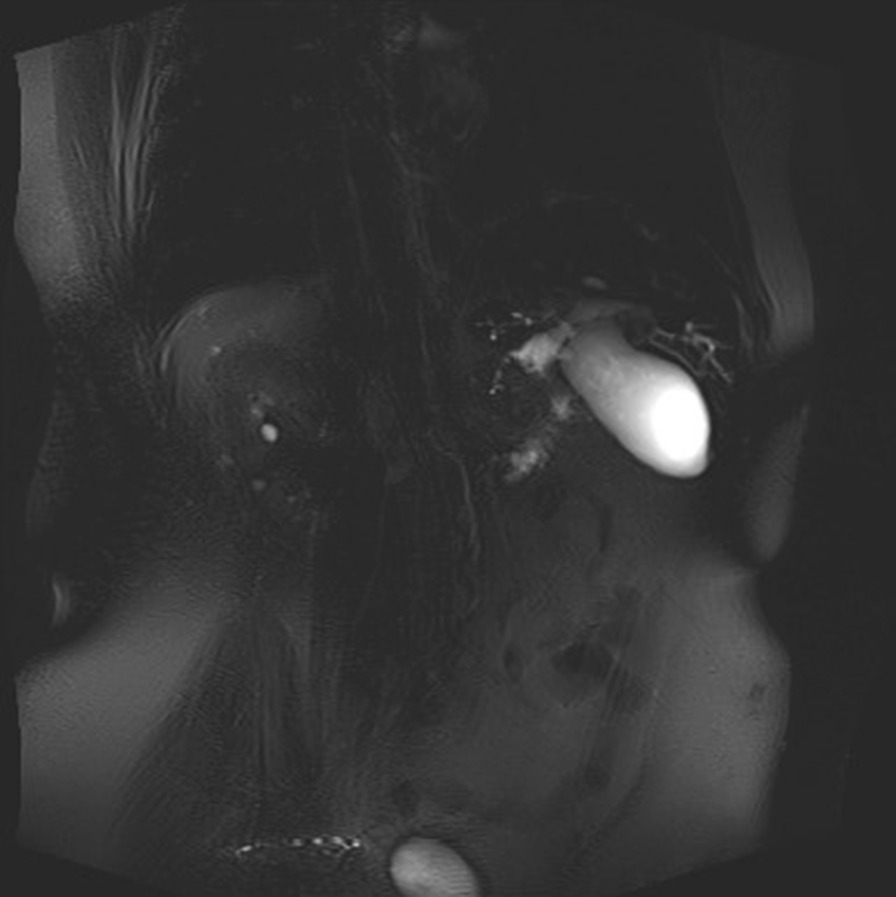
MRI- pre-stenotic common bile duct dilatation
